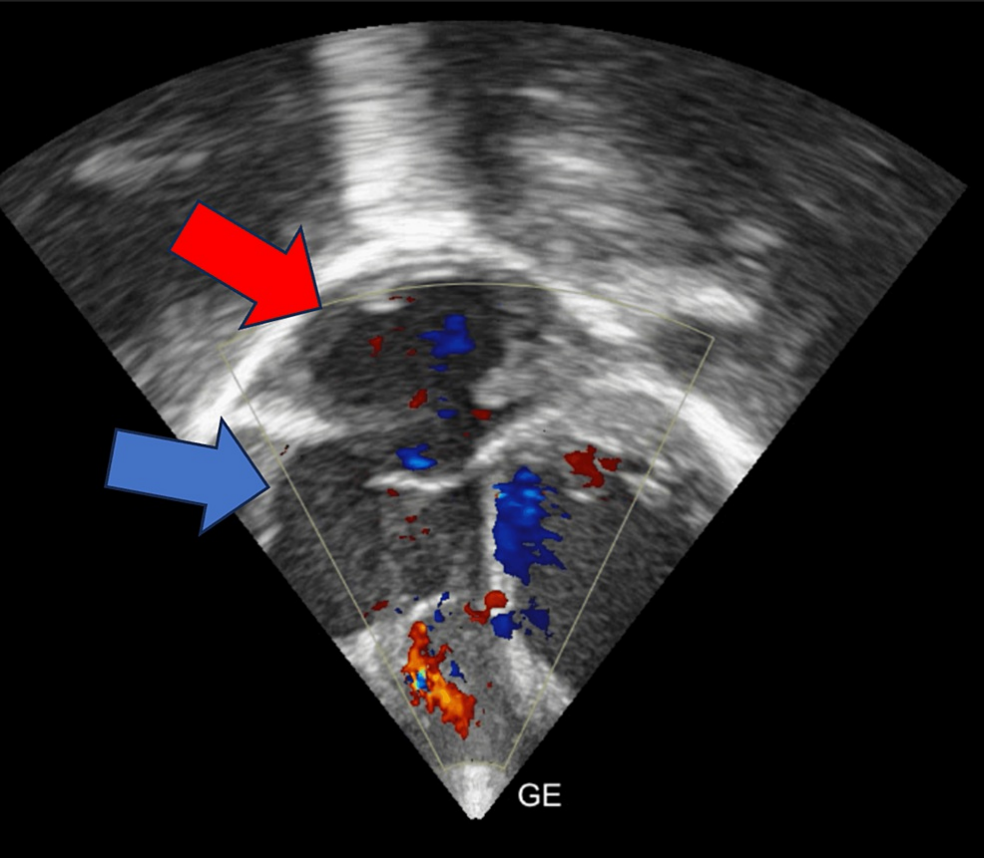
A 2D echocardiogram revealing dilated right atrium (blue arrow) and right ventricle (red arrow)
